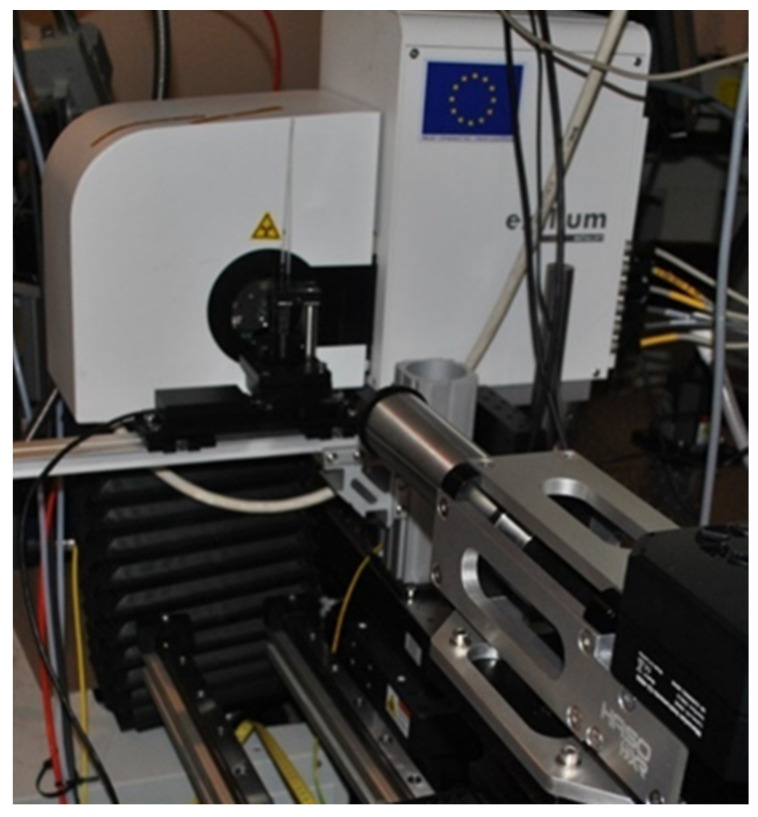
Experimental setup for phase imaging showing the Excillum X-ray source, the sample and the hard X-ray wavefront sensor (HASO HXR).
